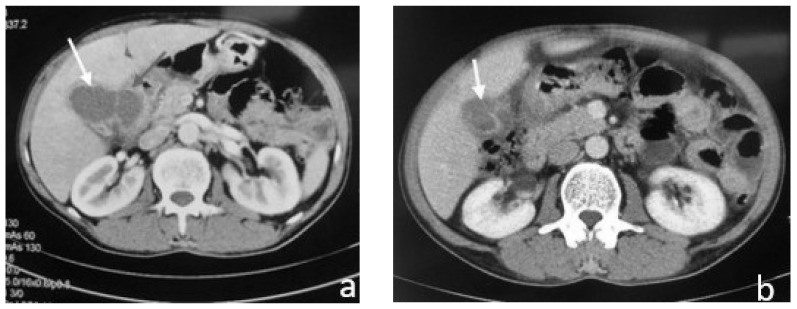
Contrast-enhanced CT shows: (a) gallbladder associated with pericholecystic fat stranding, gallbladder wall thickening, and liver abscess (white arrow); (b) gallbladder with wall thickening and a so-called “ring sign”, without central enhancement (white arrow).
